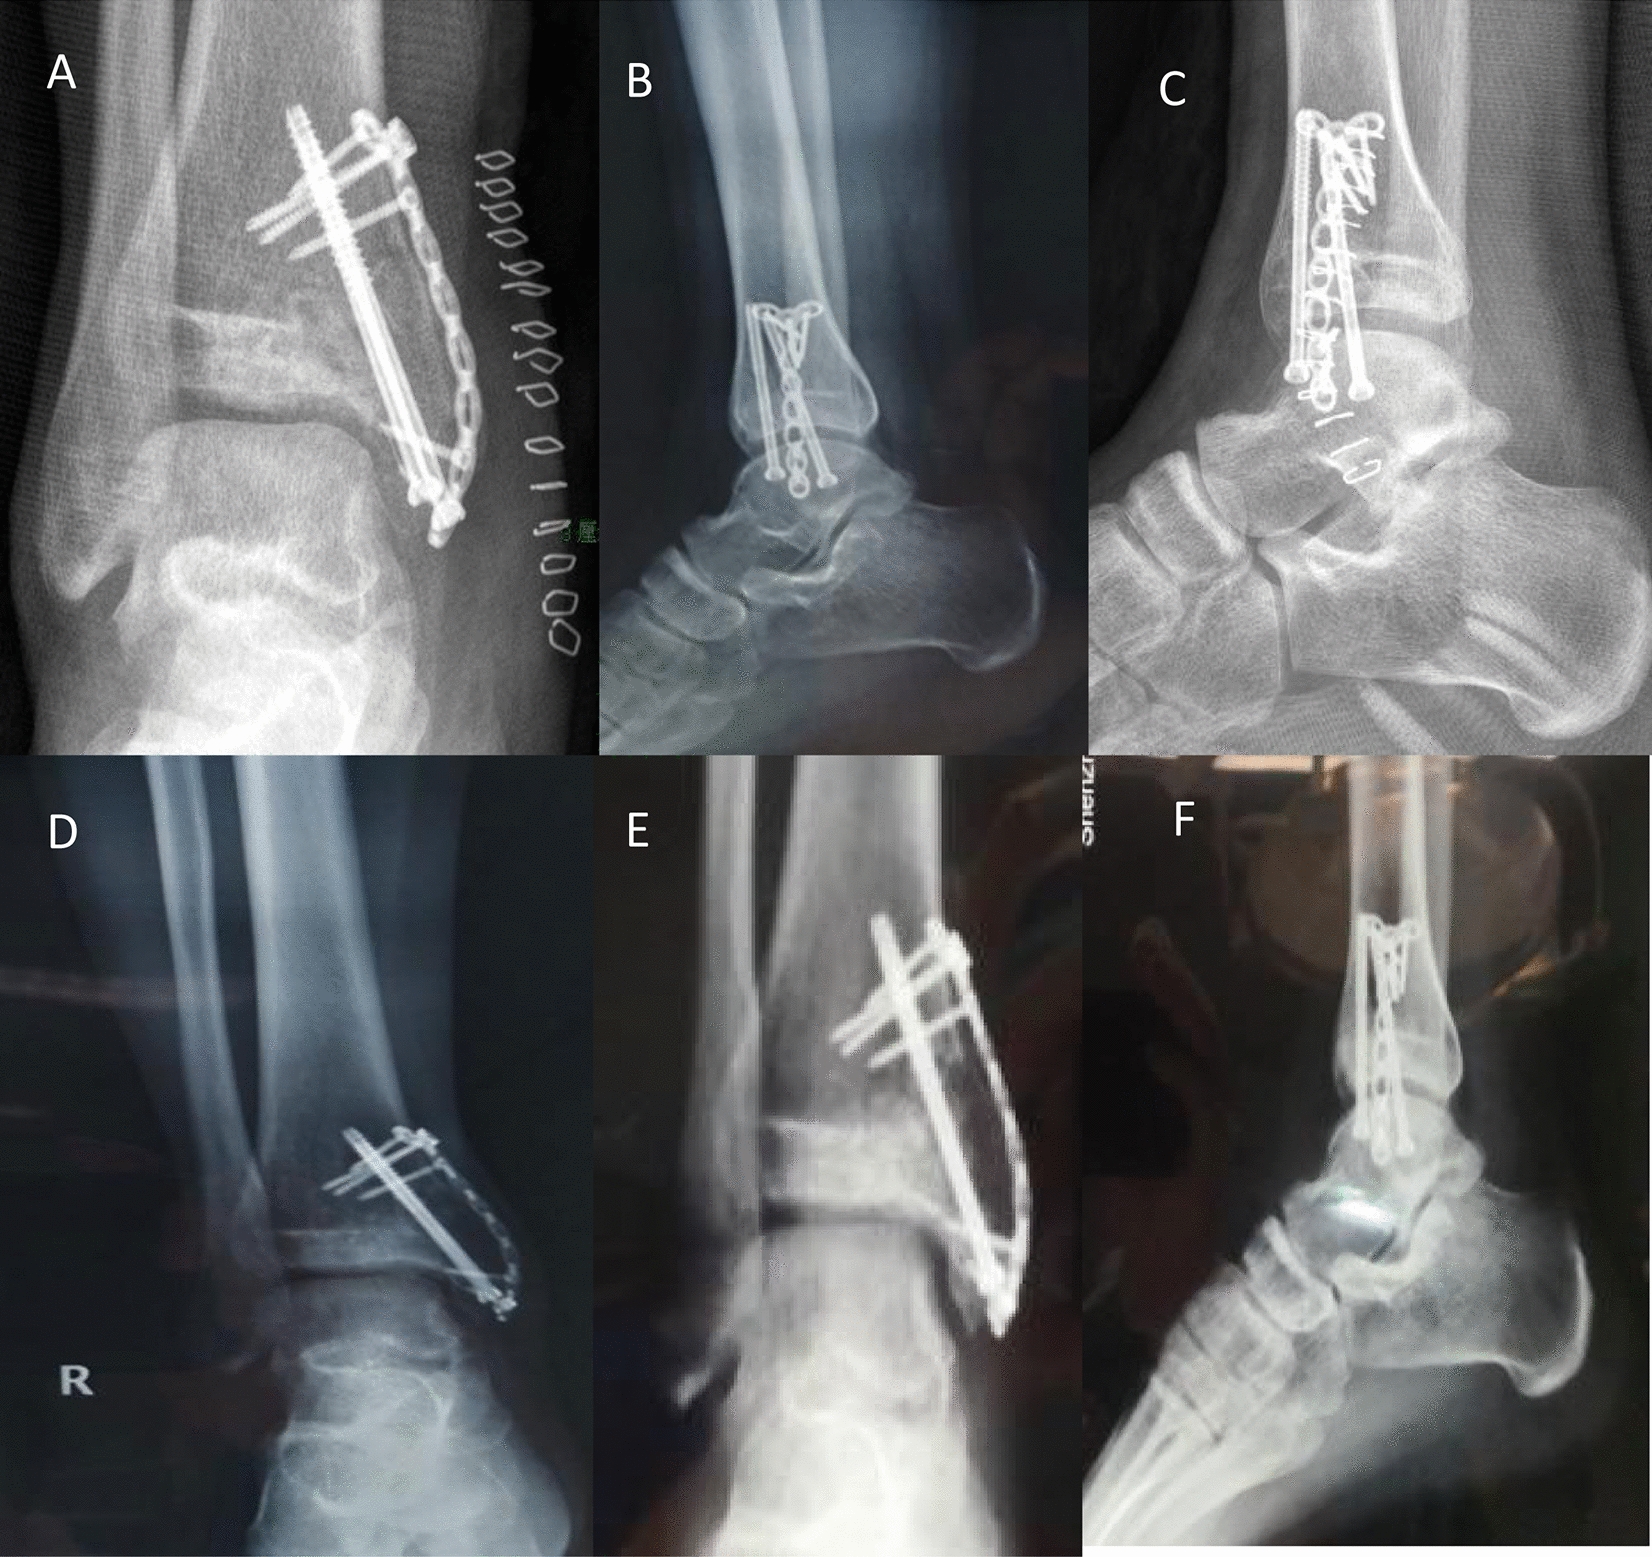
Postoperative anteroposterior and lateral X-ray examination of the ankle joint at 1 week (a, b), 1 year (c, d) and 2 years (e, f): postoperative resection of the distal right tibia lesion with bone graft fusion plate Postoperative screw fixation. The bone graft was filled and fused well. Steel plate and screw fixed firmly, no fracture, bone nonunion, etc
